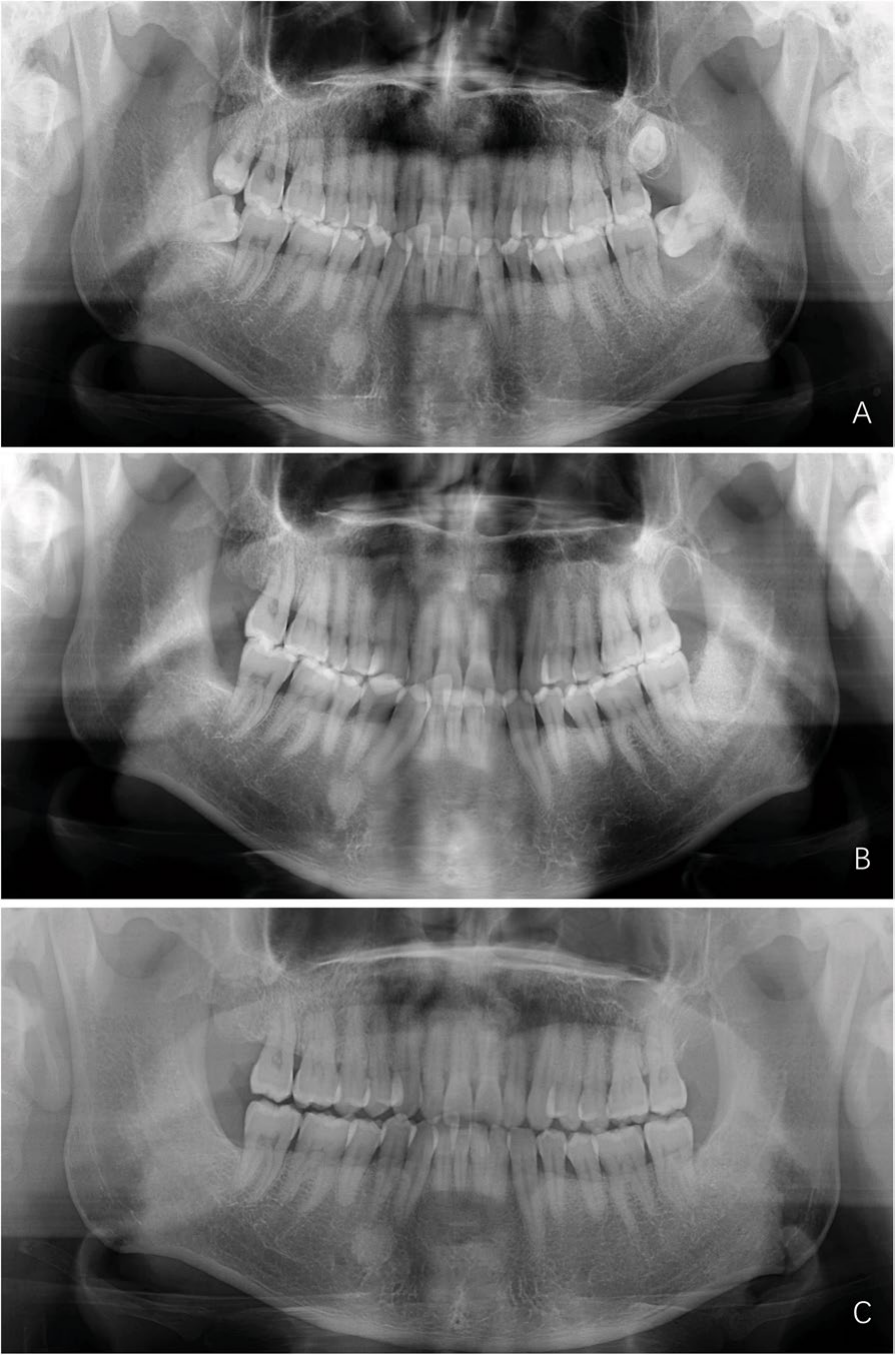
Preoperative and postoperative comparison of left third molar dentigerous cysts and repair: A Preoperative panoramic radiographs, B One-week postoperative panoramic radiographs, C Sixth month postoperative panoramic radiographs
